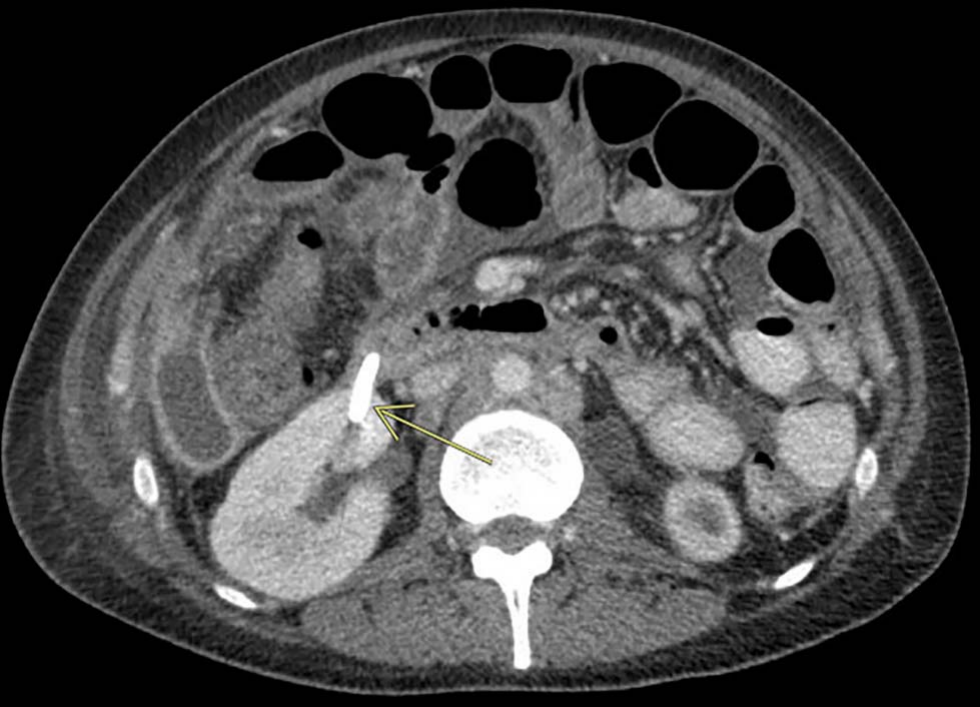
Computed tomography of the abdomen revealed that the plastic biliary stent had penetrated the posterior duodenal wall and right renal cortex, creating a 5-mm fistula.
